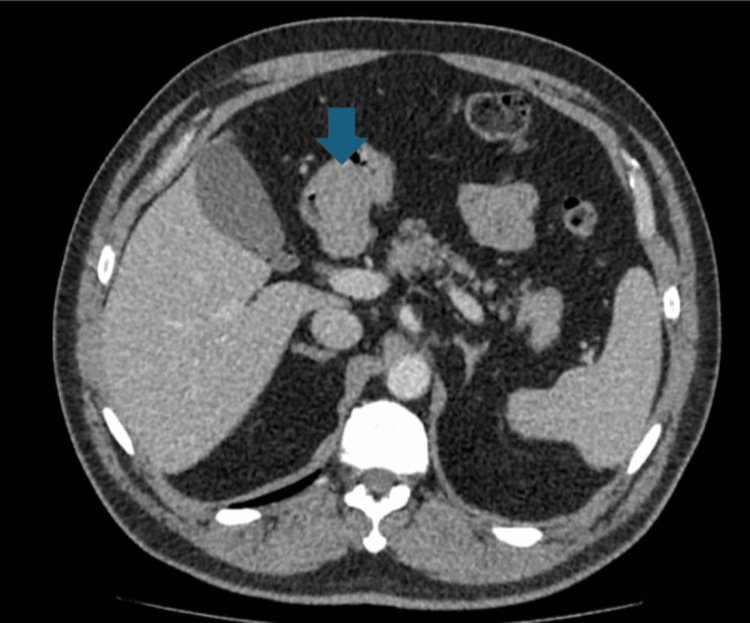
CT of the abdomen (transverse view) with the arrow showing a mass in the gastric antrum.
